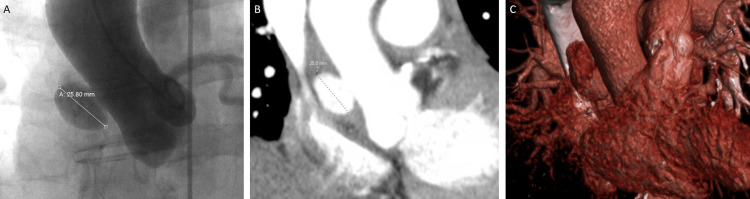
Follow-Up Imaging. Aortography (A), CT scan (B), and 3D reconstruction (C) of the chest showing an increase in the size of the ascending aortic pseudoaneurysm measuring 2.3 x 2.2 x 1.6 cm with new intramural extension of contrast.
